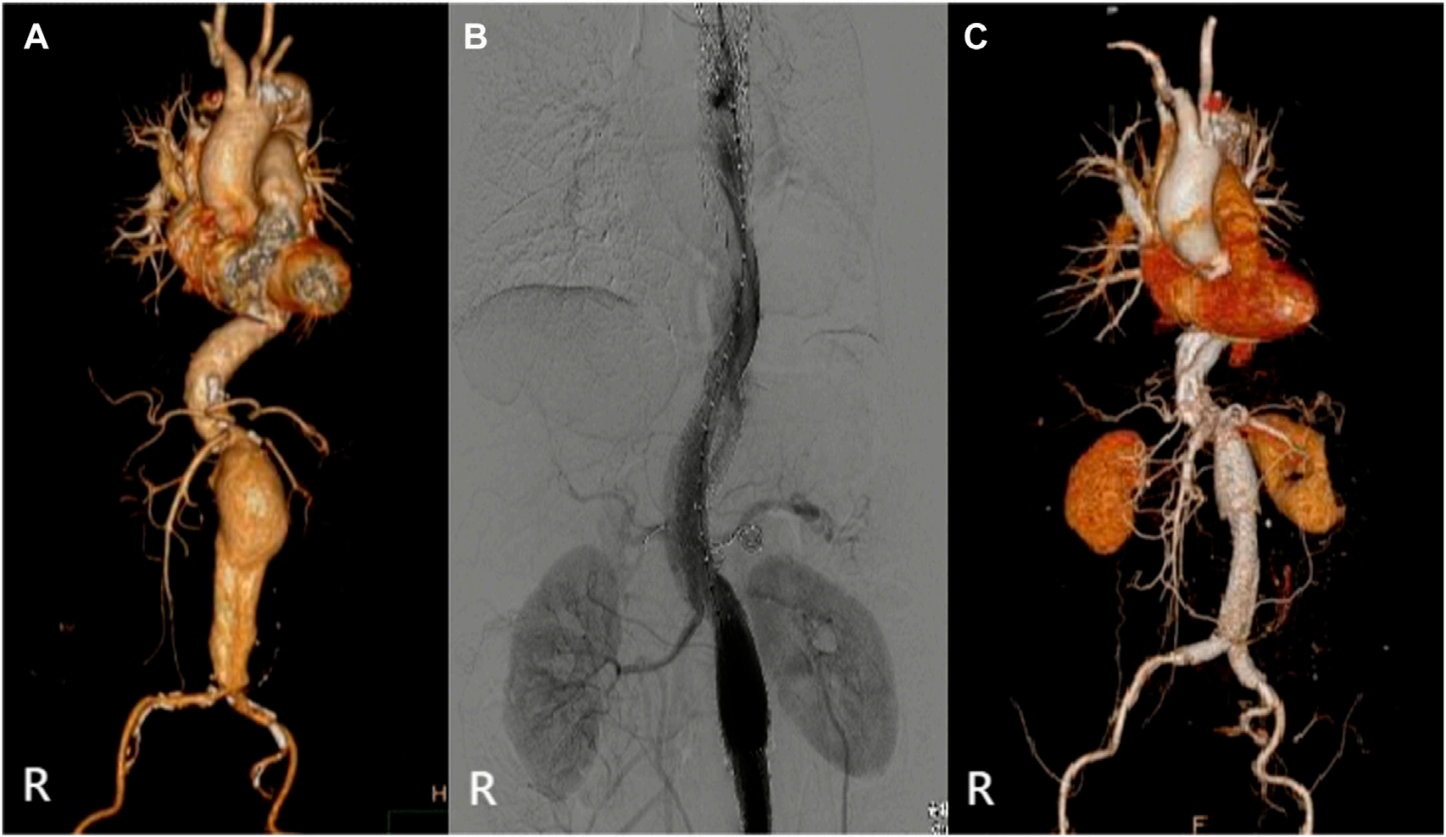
(Case 2) (A) Preoperative CTA. (B) Intraoperative imaging. (C) CTA (6th month).
